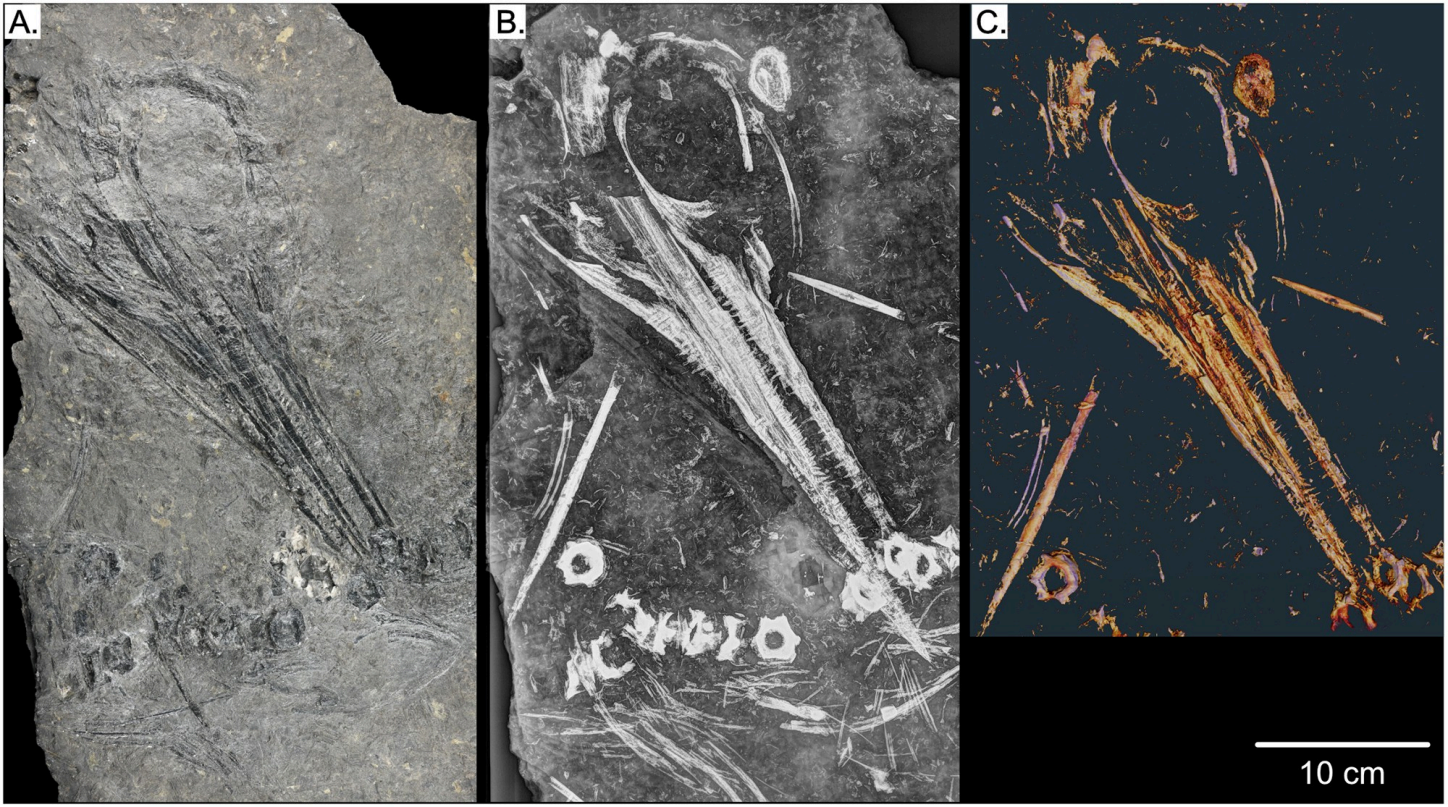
Method comparison on the skull of PMO 219.250. A. Photograph (visual observation). B. Radiograph. C. Computed Tomography scan.
